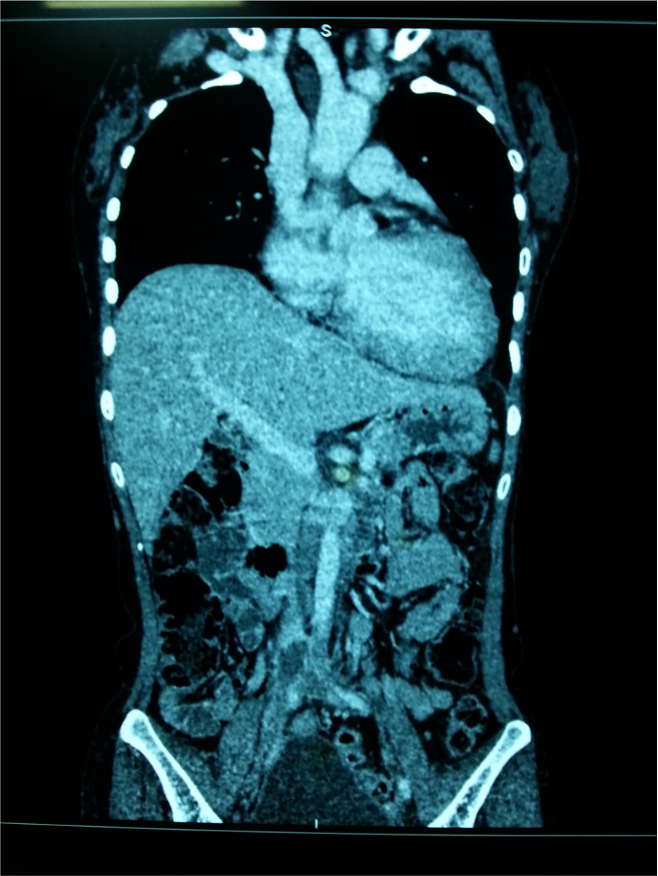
Computed tomography cavogram. A 4.8cm segment of thrombosis of the inferior vena cava, extending from just below the renal vein up to the bifurcation.
